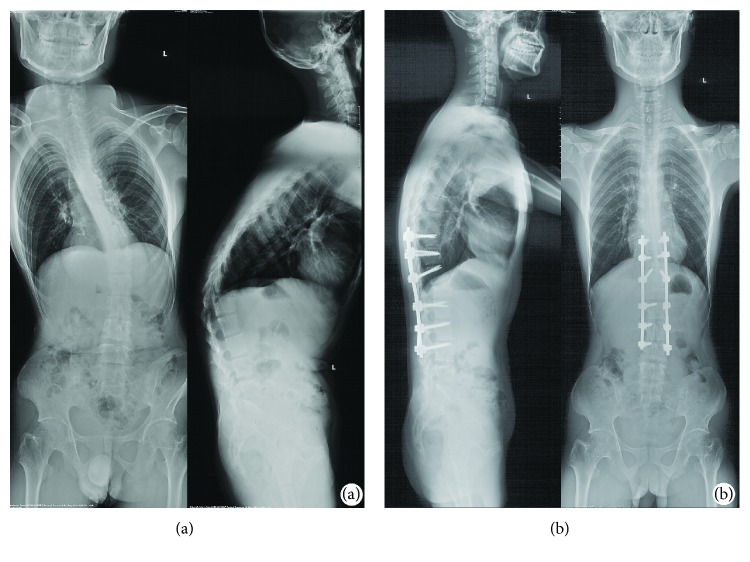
The full-length spine radiographs of a patient were obtained before surgery and one week after surgery. (a) Before surgery, the apical (kyphosis) vertebra was L1, with thoracolumbar kyphosis (T10 to L2) of 52°. The coronal Cobb angle between T9 and L3 was 56°. (b) Vertebral column decancellation was applied at L1, and the spine was fused from T10 to L4 with pedicle screws and a rod system. The thoracolumbar kyphosis and scoliosis were corrected to 11° and 12°, respectively.
